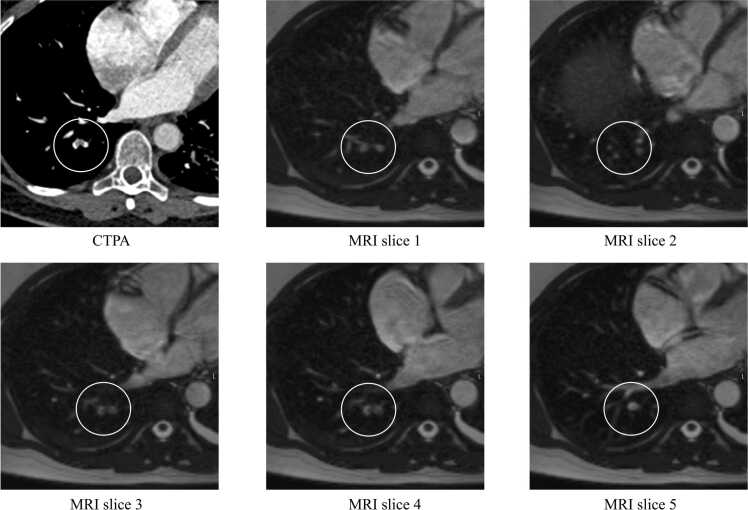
As shown in these figures the embolus is only visible in slices 3 and 4, that is, in only 2 out of the 5 MRI images from the same slice position.2.Following the vessels while scrolling through the stacks feels subjectively easier and more like CT stacks, according to the radiologists analyzing the studies, thereby making it more familiar. This could be explained by the number of images in the MRI stacks, which are approximately the same as in CTPA.
